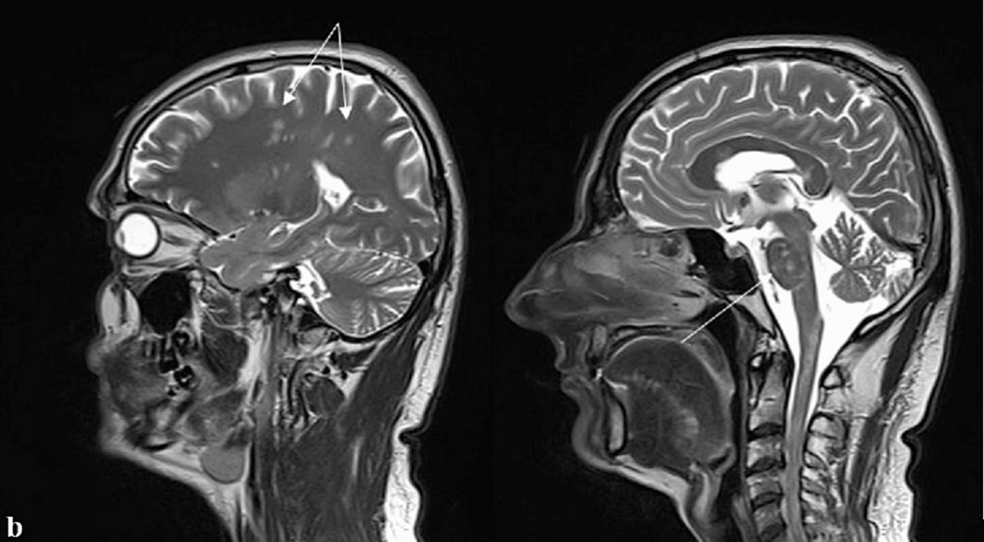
Sagittal T2 MRI of the brain showing multiple hyperintense lesions in the pons and subcortical white matter MRI: magnetic resonance imaging
